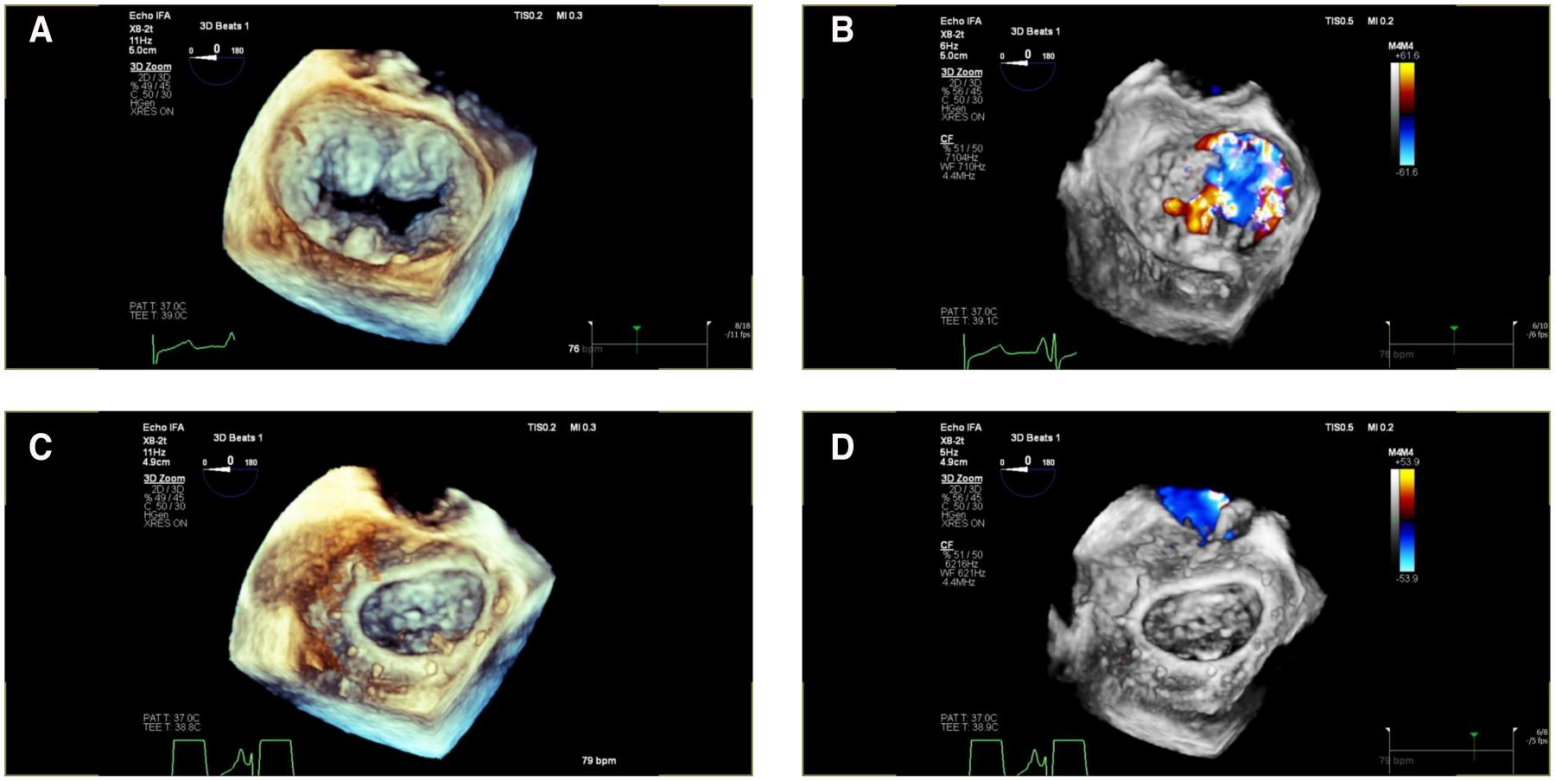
Three-dimensional transoesophageal echocardiography of the mitral valve in a patient with Barlow’s disease before (upper panels) and after mitral valve repair with Memo 4D annuloplasty ring (lower panels): en-face views of the mitral valve from the atrial perspective (A–C), colour Doppler (B–D).
